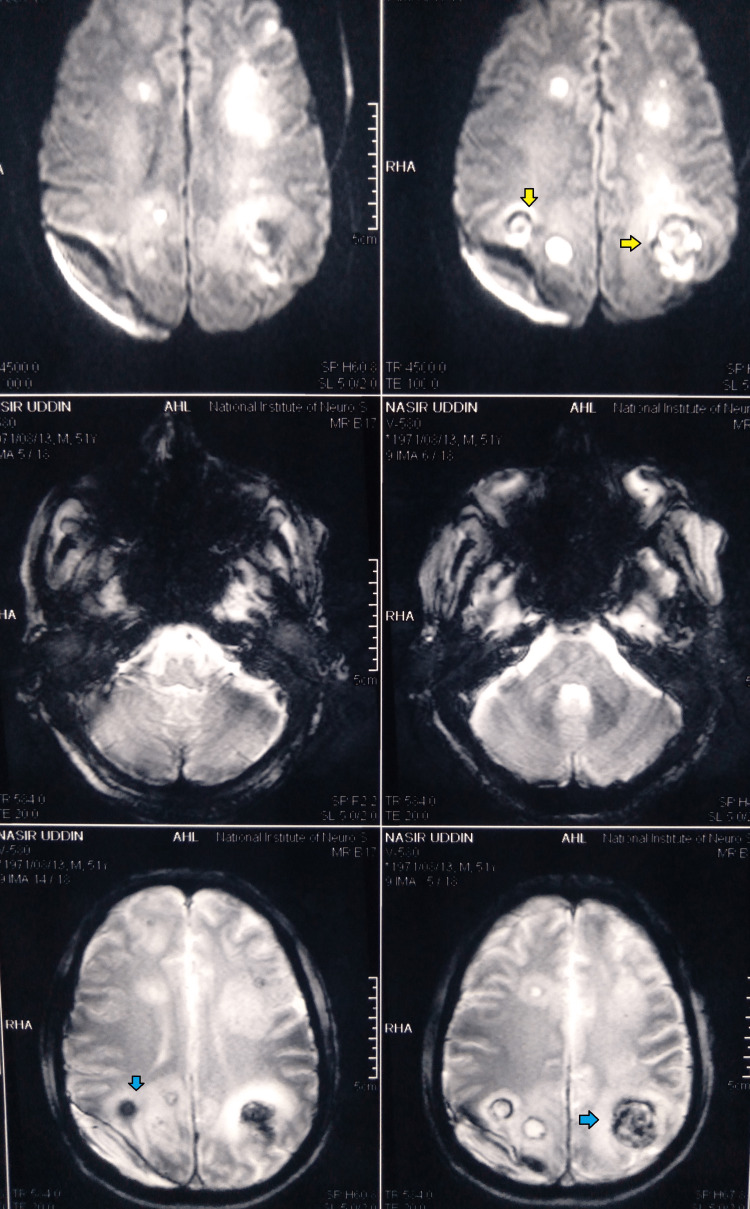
MR perfusion scan shows diffusion restriction in DWI (yellow arrow) and blooming in GRE sequence (blue arrow) DWI- diffusion-weighted imaging; GRE- gradient recalled echo
